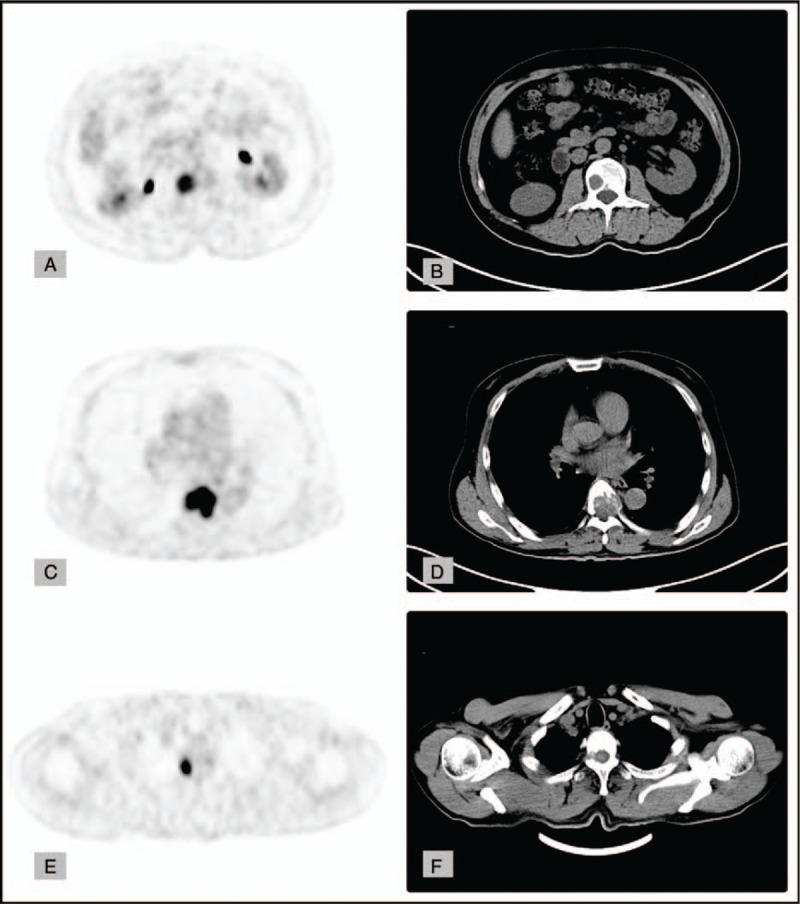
A–F, Positron emission tomography-computed tomography shows vertebral metastasis and increased fluorodeoxyglucose uptake in multiple nodules in the liver and adrenal gland. The location of the metastases is along the lines of the craniospinal venous system.
